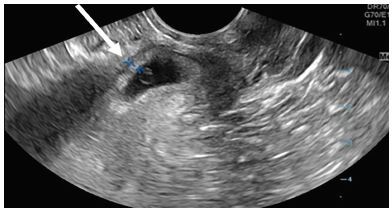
Transvaginal ultrasound view of uterus in sagittal plane showing cesarean scar ectopic pregnancy. Myometrium thickness of anterior uterine segment (arrow) measures 0.35 centimeters, which meets diagnostic criteria for ectopic pregnancy.^6^
